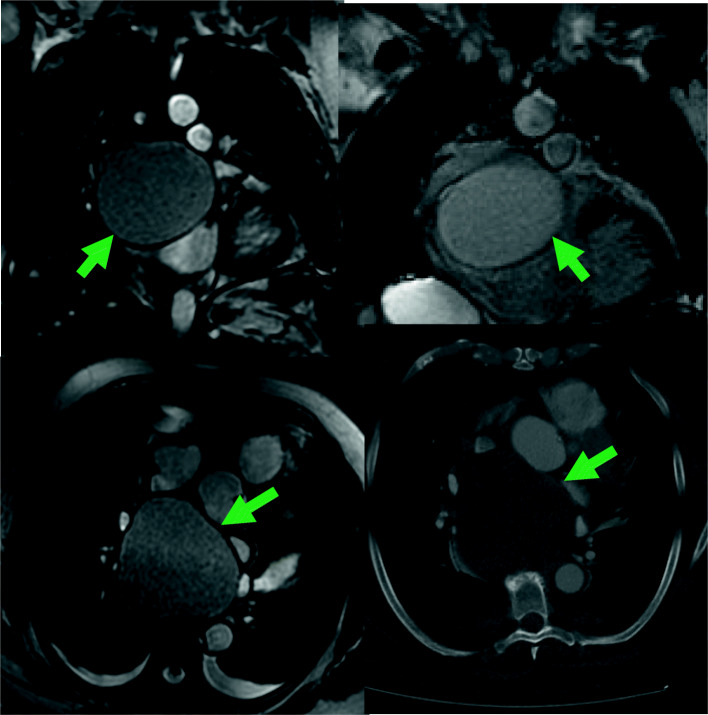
Cardiac MRI. Green arrows represent the dimensions and localization of the intrapericardial mass
